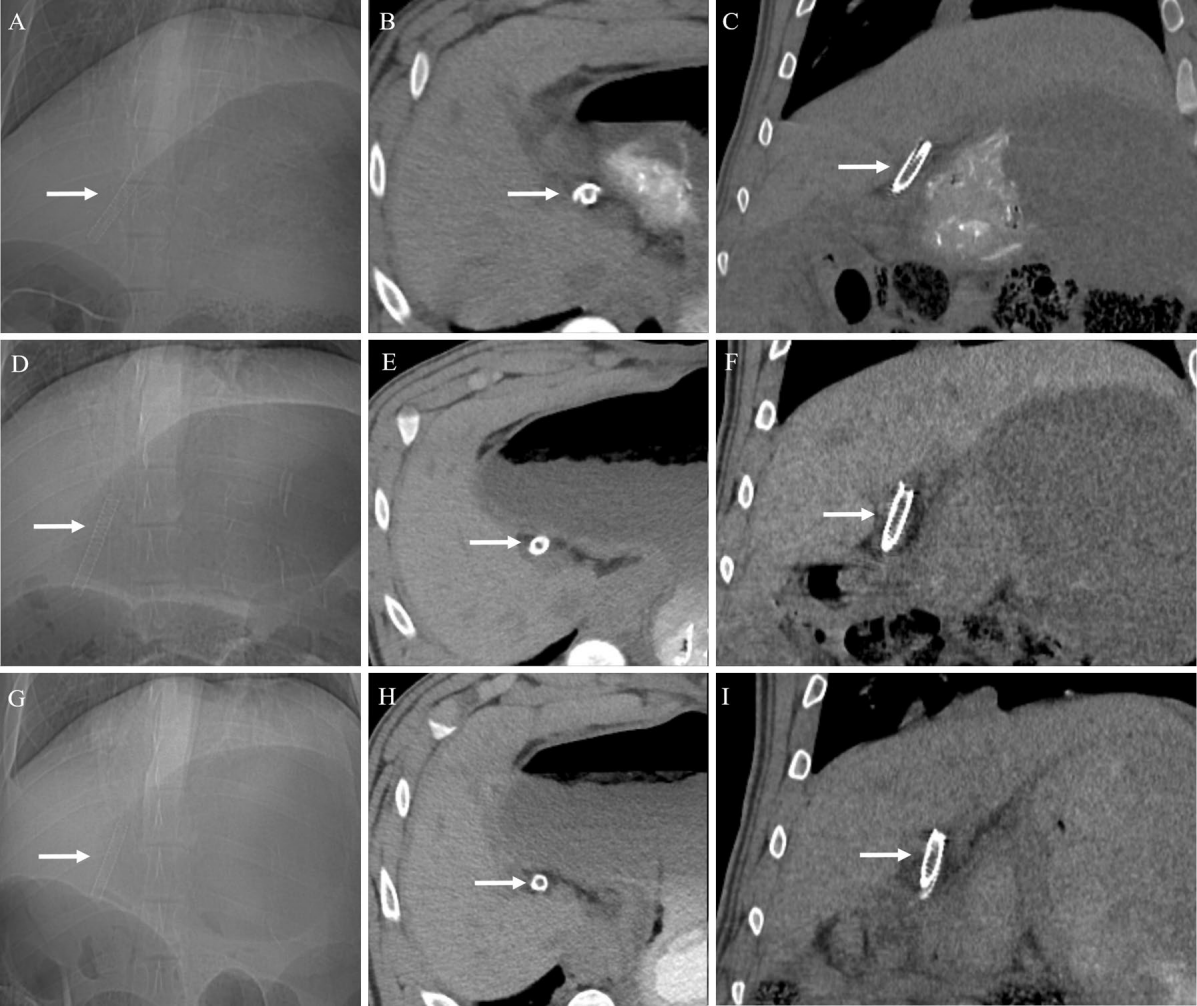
Representative images of 1-month (A–C), 2-month (D–F), and 3-month (G–I) follow-up CT scans to evaluate the status of biodegradable stents. The scout images (A,D,G), precontrast axial images (B,E,H), and coronal reconstruction images (C,F,I) show a radiopaque stent in the common bile duct without complications.
